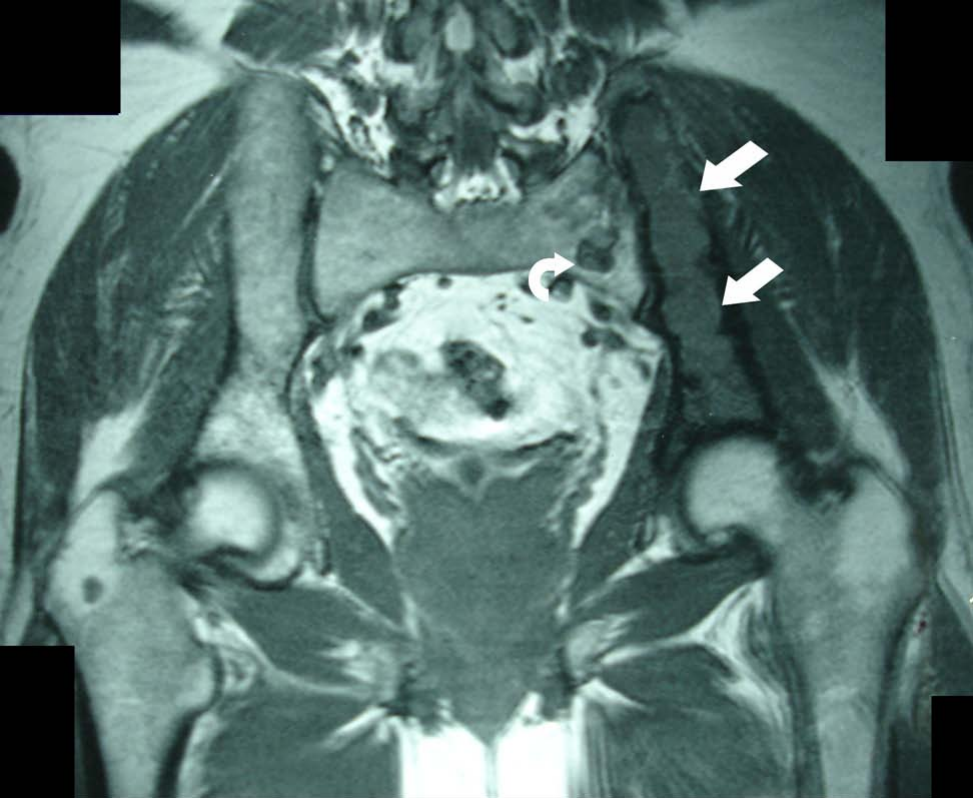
Coronal T1-weighted MRI demonstrates decreased signal at the left iliac bone (arrows) and at the left sacral wing (curved arrow).
